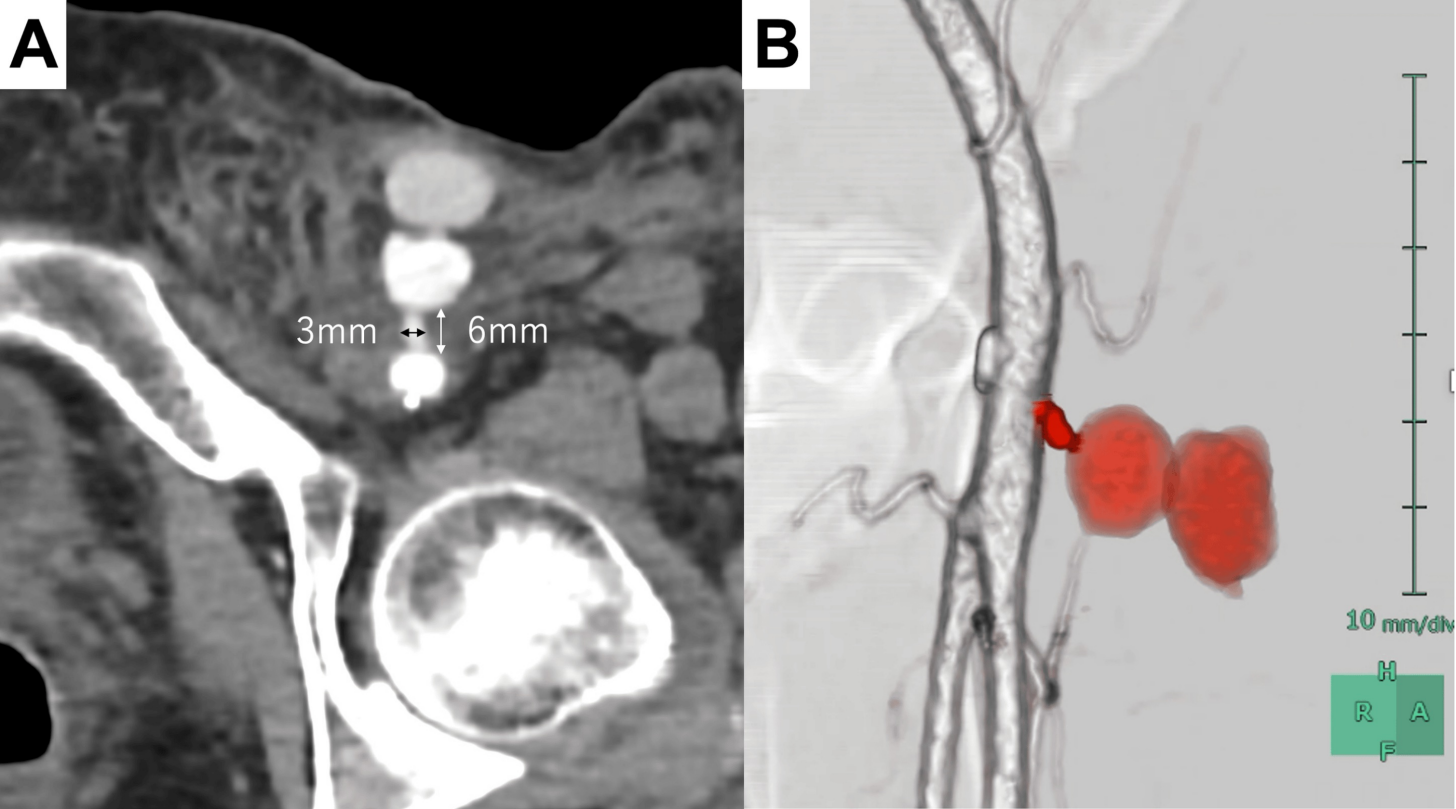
Contrast-enhanced computed tomography images of the left femoral artery pseudoaneurysm A: Axial image; B: Volume-rendering image created using SYNAPSE VINCENT (Fujifilm, Tokyo, Japan). The red color indicates the tract and pseudoaneurysm, and the white color shows the left femoral artery. Colors were added during postprocessing for better visualization of the anatomical structures.
